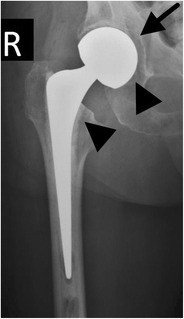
Anteroposterior (AP) radiograph. Cemented unipolar hemiarthroplasty. The femoral stem with the fixed head (*arrowheads*) articulates with the native acetabulum (*arrow*)
